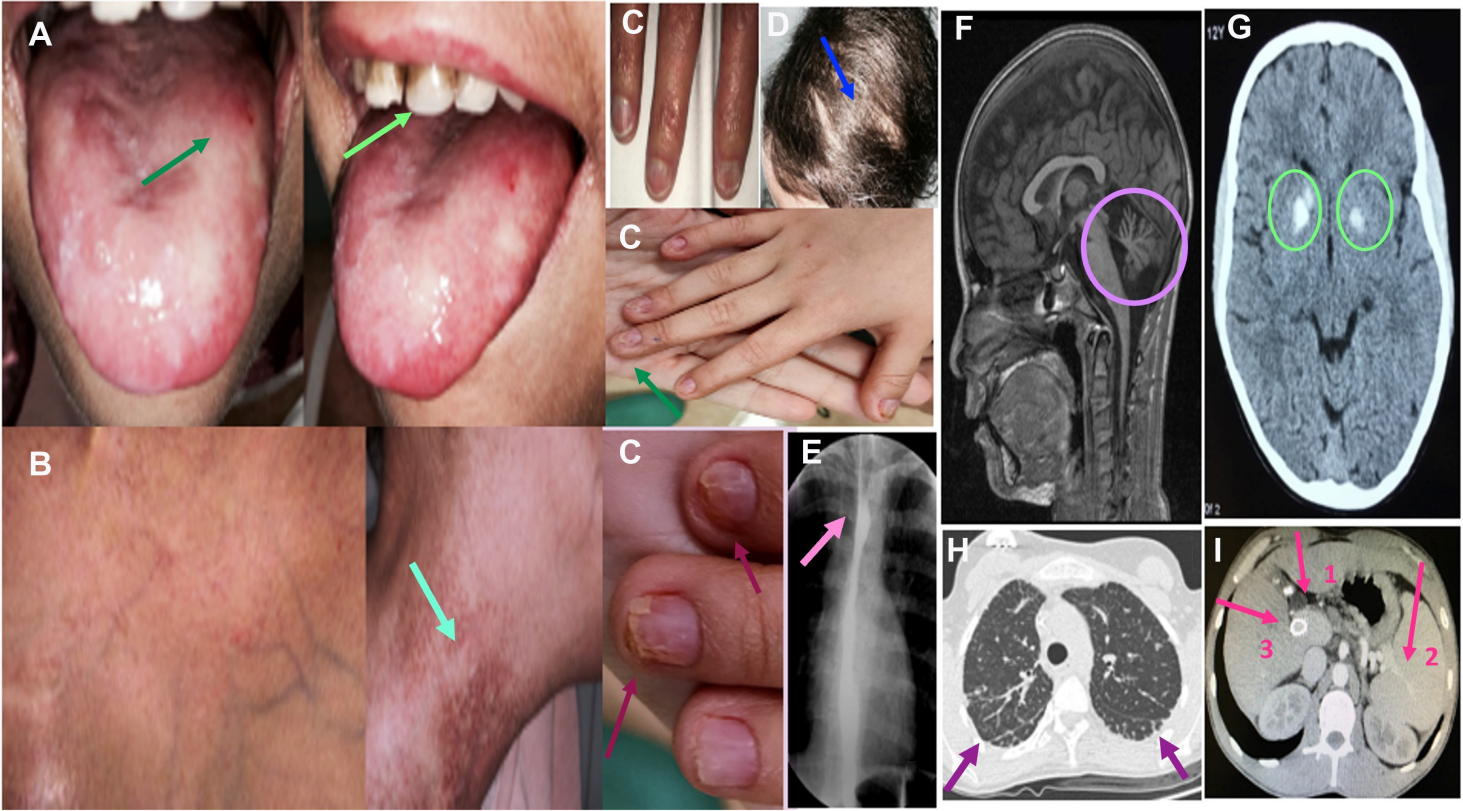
Clinical and radiological findings in DC. (A) Patient 5: oral leukoplakia and tooth involvement. (B) Patient 5: reticular pigmentation of the neck. (C) Patients 4, 9 and 10: nail dystrophy. (D) Patient 9: scarce and sparse hair. (E) Patient 9: Esophageal stricture. (F) Patient 3: Brain MR shows cerebellar hypoplasia in a patient diagnosed with Hoyeraal-Hreidarsson Syndrome. (G) Patient 2: Cranial CT shows calcifications of the basal ganglia in a patient with Revetz Syndrome. (H) Patient 3: Axial section of the chest CT showing signs of incipient pulmonary fibrosis with subpleural lines predominantly basal and heterogeneous distribution. (I) Patient 8: Abdominal CT showing signs of severe liver fibrosis: portal vein dilatation (arrow 1) and secondary splenomegaly (arrow 2). Transjugular intrahepatic portosystemic shunt (TIPS) is also shown (arrow 3).
